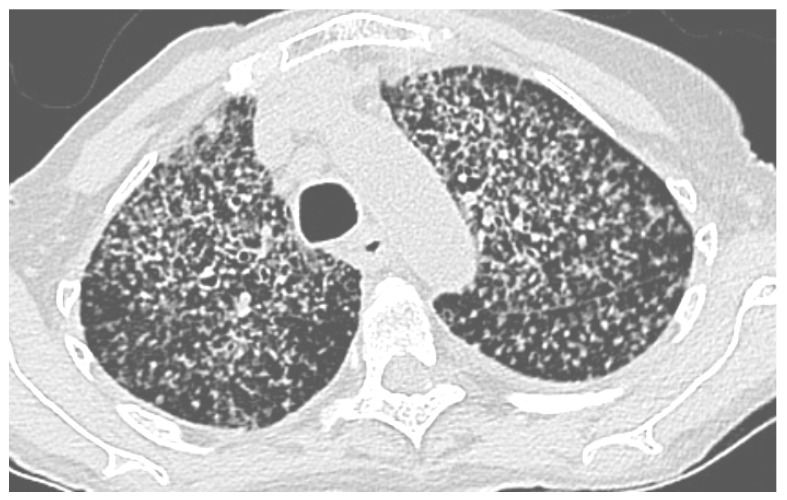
Chest CT scan, miliary histoplasmosis in 91-year-old woman occurring after cleaning droppings, particularly those of bats, in a confined place without protection. She unfortunately died despite treatment. Scale bar: 25%.
